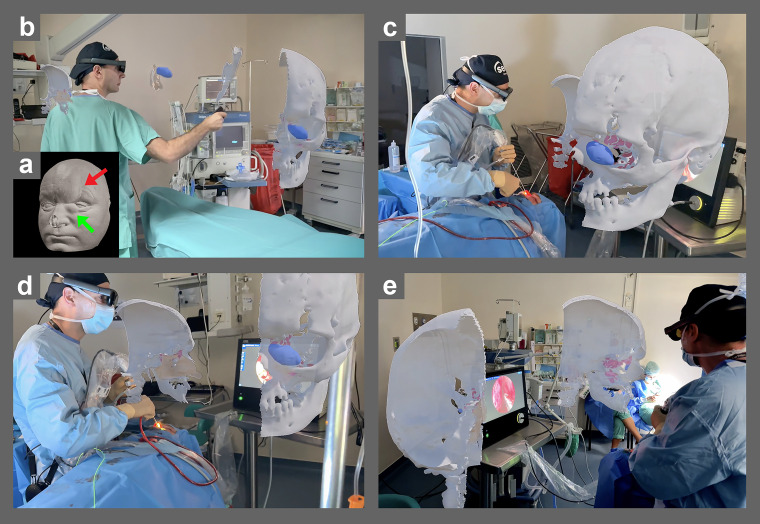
MR-supported operation theatre. (a) face 3D reconstruction obtained from CT (red arrow-groove after maxillofacial trauma, green arrow—massively enlarged lacrimal sac; left globe dislocated inferiorly; left ptosis); (b) operation theatre setup for MR-supported surgery; (c–e) MR-assisted EnDCR.
